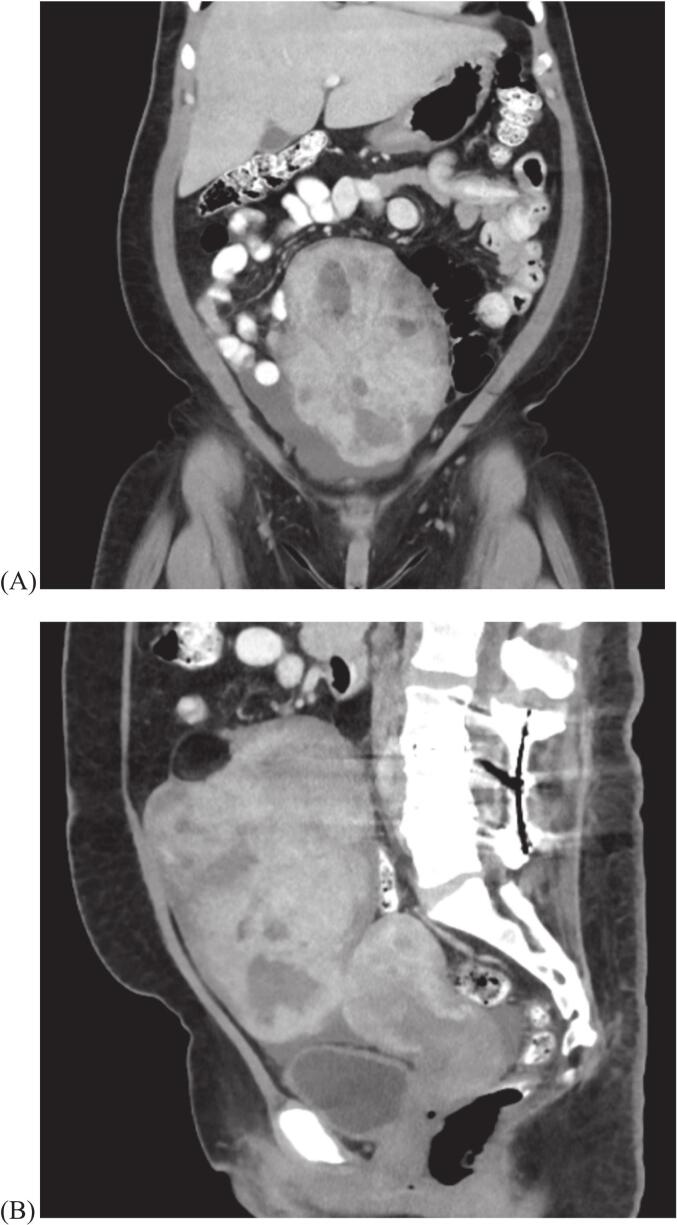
(A) Coronal Computed Tomography scan showing a heterogeneous, predominantly solid, right adnexal mass. (B) Sagittal Computed Tomography scan showing a heterogeneous, predominantly solid, right adnexal mass with fatty subcapsular component.
